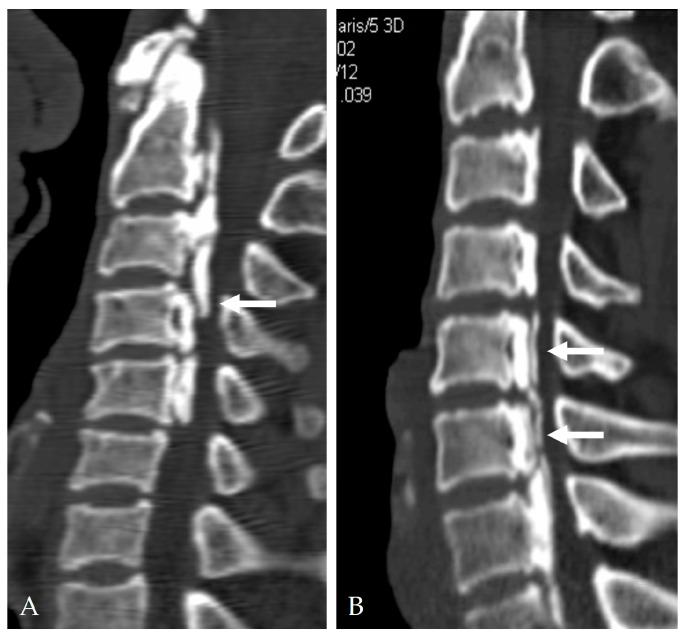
2-dimensional sagittal reconstructed CT showed ‘double-layer’ sign (white arrows) (A,B).
